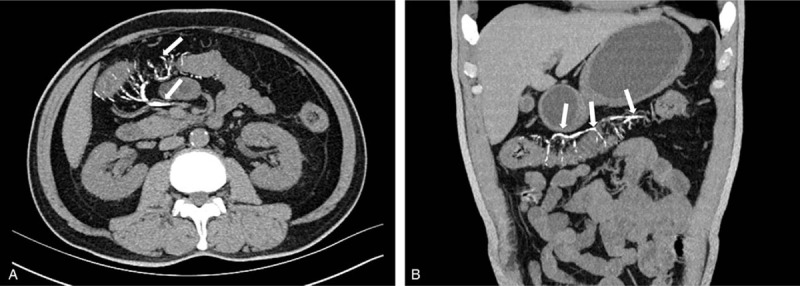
Abdominal CT plain scan. (A-B) Axial images and coronal reformatted images showed colonic wall thickening involving the ascending colon to the transverse colon, with characteristic threadlike calcifications (white arrow) in the subserosal and mesenteric veins.
